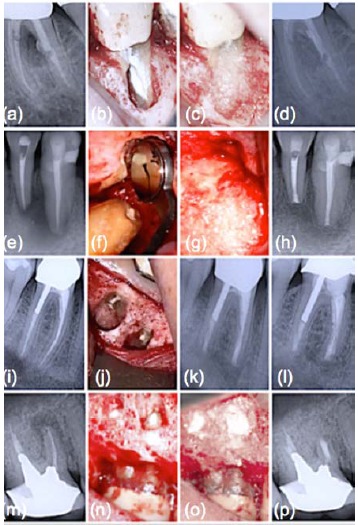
Clinical application of B-G during endodontic surgery. (a) Intraradicular post protruding through a perforation is noted radiographically; (b) Post reduction followed by root repair; (c) B-G placed into the surgical crypt prior to closure; (d) At one-year follow-up, resolution of furcal radiolucency; (e) Preoperative radiological situation; (f) Completed root-end filling; (g) B-G embedded in the bone cavity; (h) Resolution of radiolucency and reattachment of periodontal ligament at one-year control; (i) Preoperative periapical x-ray; (j) Retrofilling placed; (k) Immediate post-operative x-ray following apicoectomy with concomitant B-G grafting; (l) One-year follow up radiograph shows complete healing; (m) Preoperative periapical radiograph; (n) Terminated retrograde filling; (o) Intraoperative image showing B-G in place to fill in the bony cavity; (p) Radiological situation one year after surgery representing complete resolution of the periapical lesion.
